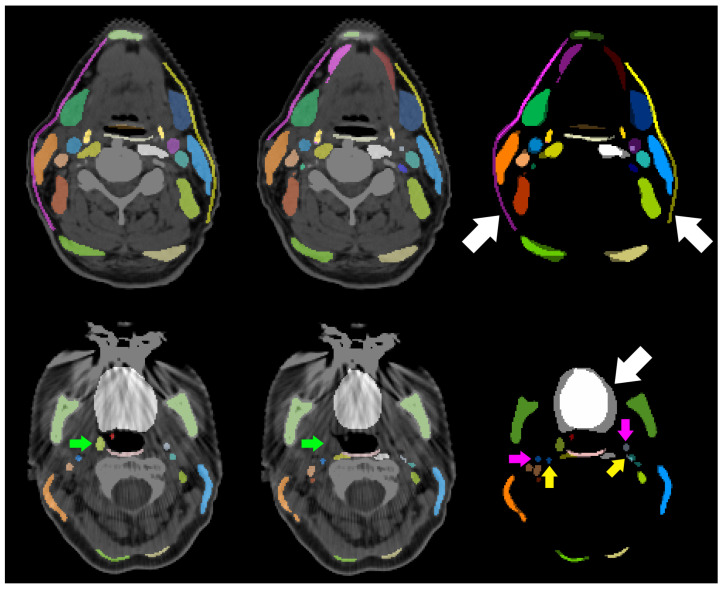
CT slices of two different patients with contours generated manually (left), contours generated by our trained nnU-Net models (middle), and the comparison of both contours without CT slice (right). White arrows indicate large deviations between both contours in the platysma (top row) and the tongue (bottom row). Deviations in the segmentations of the internal carotid artery are indicated by pink arrows (manual labels) and yellow arrows (predicted labels). The right tonsil (green arrow) is not visible.
